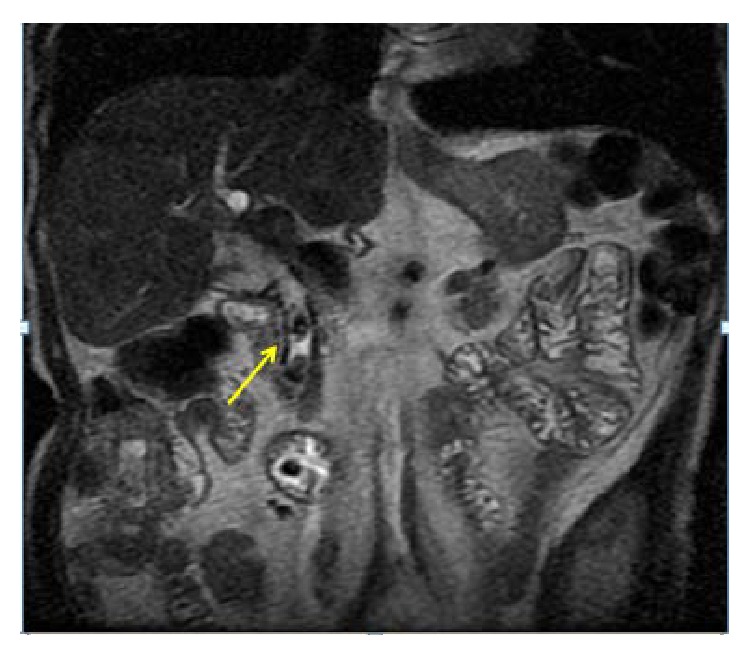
Magnetic resonance* cholangiopancreaticography showing obstructive biliary processes associated with stent dysfunction of the extrahepatic and common bile duct. Intraluminal irregular images (yellow arrow) of low signal intensity on T2-weighted sequences consistent with bile stones are observed; these lead to moderate dilation of the intrahepatic bile duct.
